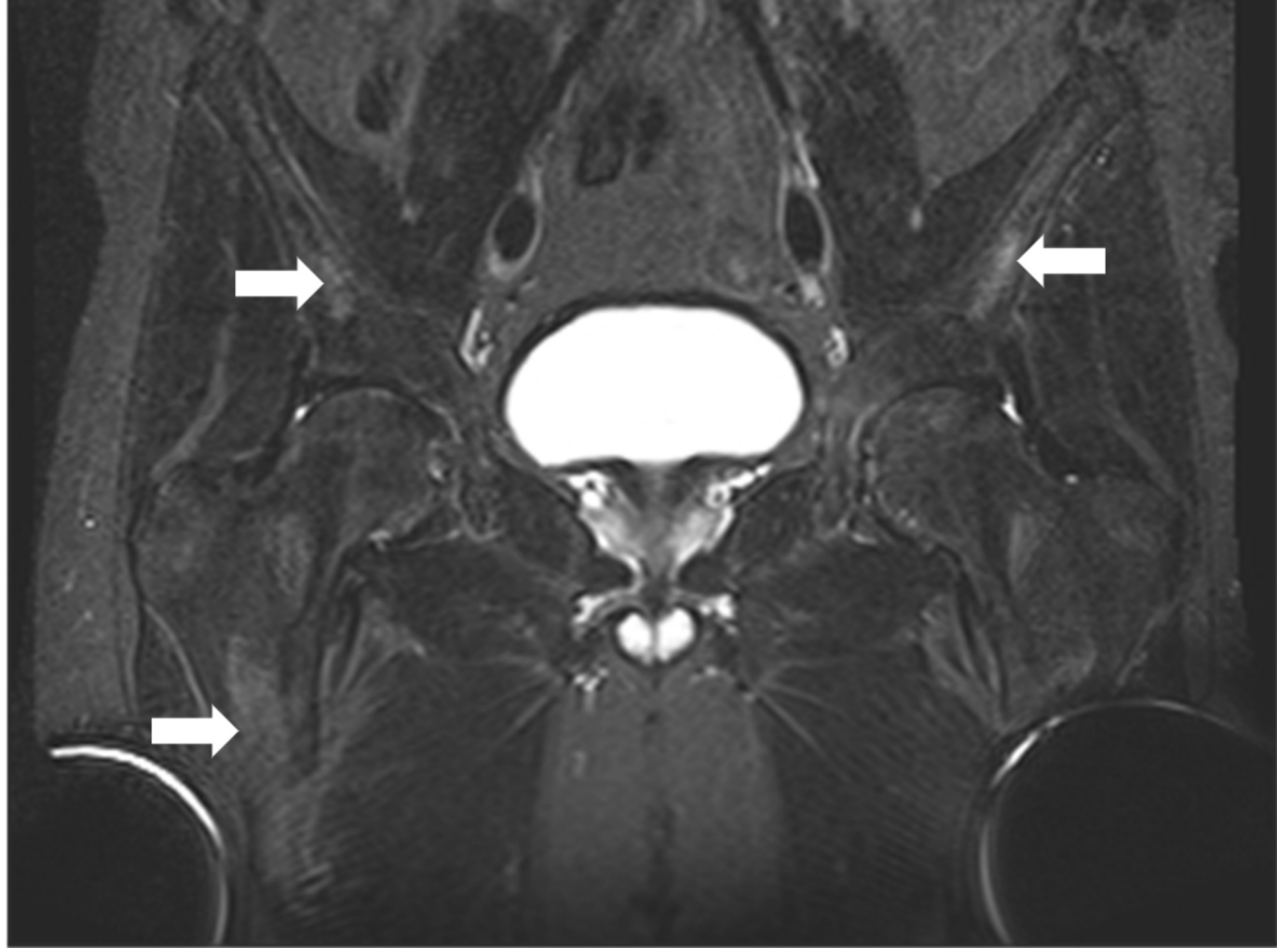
Pelvic magnetic resonance imaging Diffuse short tau inversion recovery (STIR) hyperintensities throughout the osseous pelvis concerning for metabolic bone disease and possible osteonecrosis.
